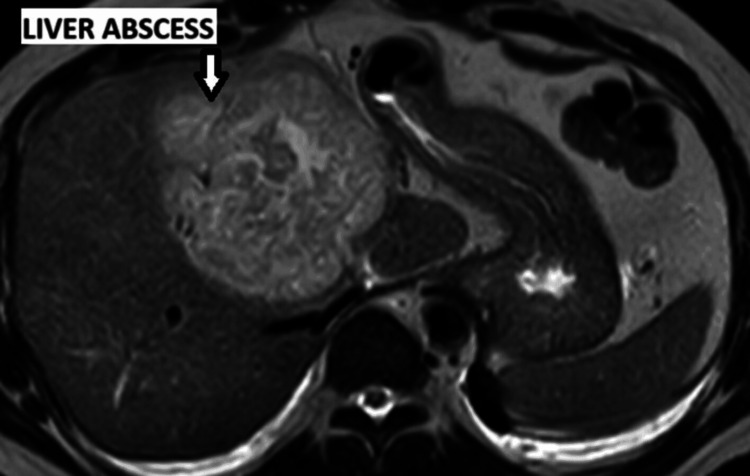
MRI of the abdomen showing a large liver abscess
